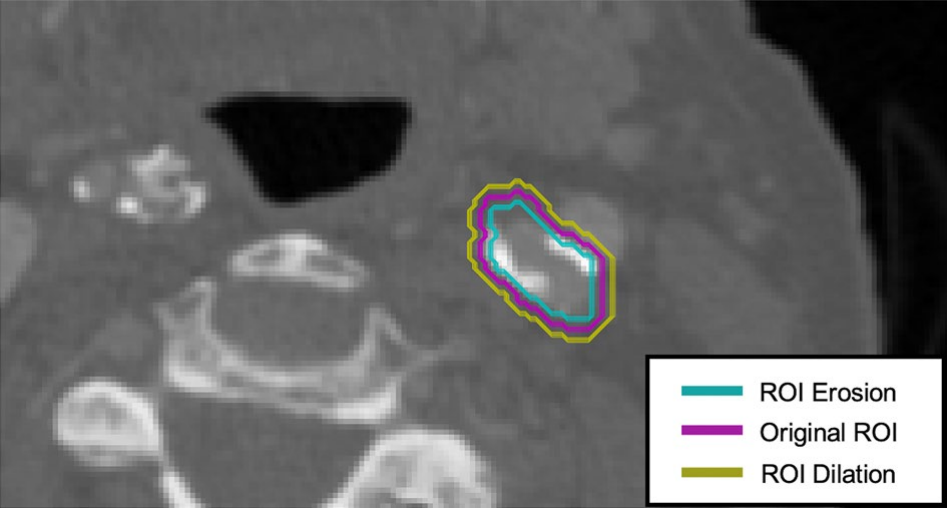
ROI segmentation and perturbations. Carotid CTA images were manually segmented to delineate the carotid artery. The original ROI was subjected to morphological operations: erosions and dilations aimed to assess the robustness of radiomic features to perturbations in image segmentation.
